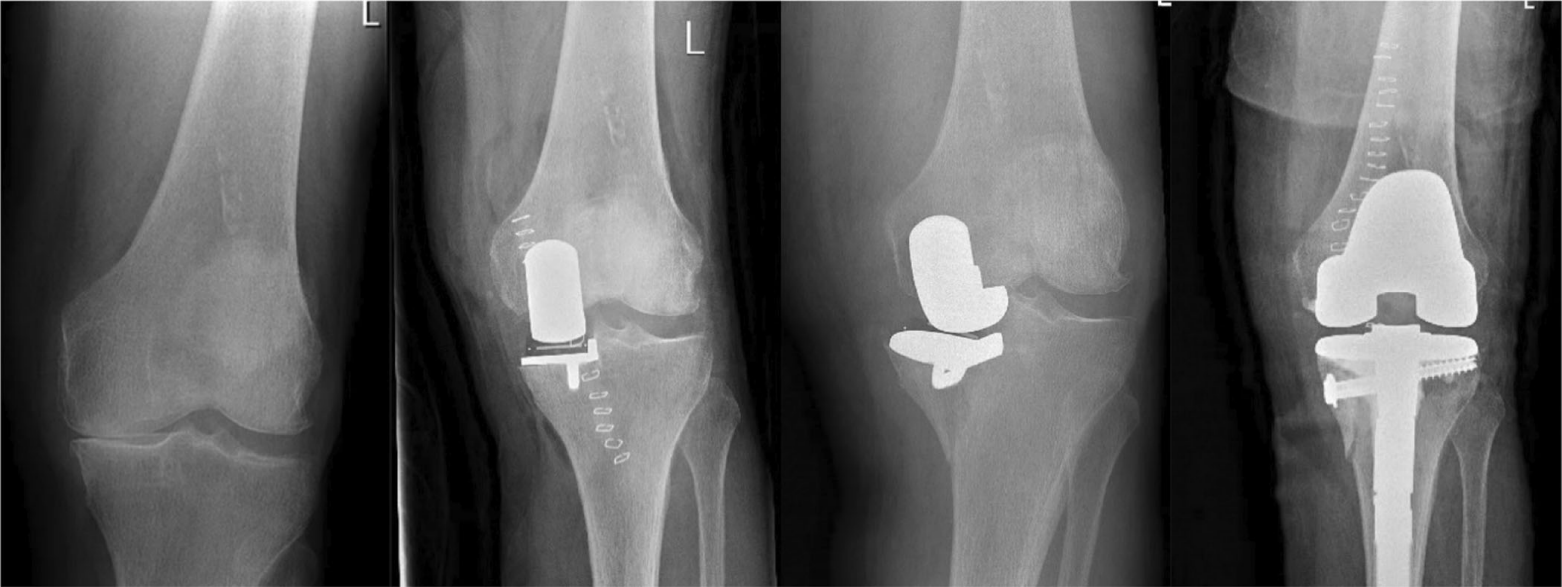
Preoperative radiograph shows bone‐on‐bone medial osteoarthritis. The post‐operative radiograph suggests a deep vertical tibial saw cut, leading to a periprosthetic fracture of the tibial component. This patient was revised with a tibial revision stem and screw augmentation.
